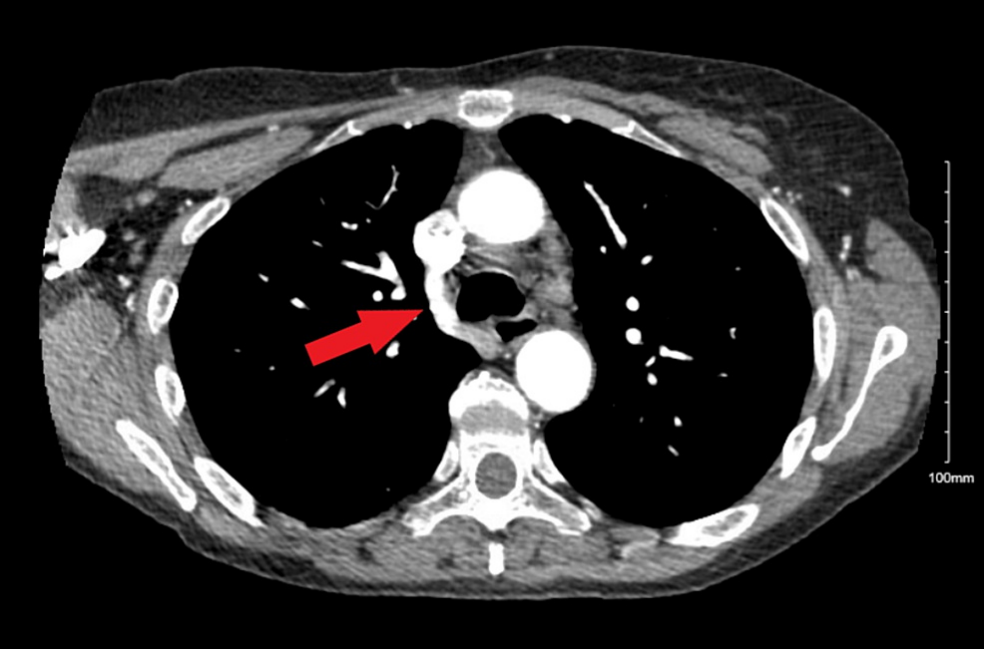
CT angiogram showing an aberrant right subclavian artery
